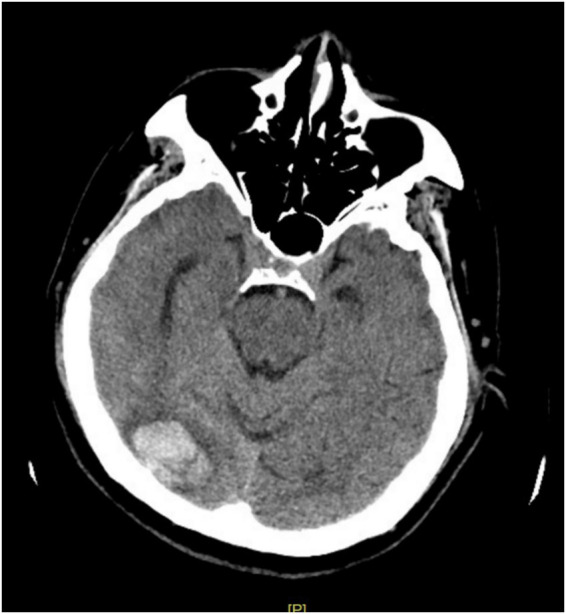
Emergency cranial CT scan (May 24, 2025) reveals a hemorrhage in the right occipital lobe.
